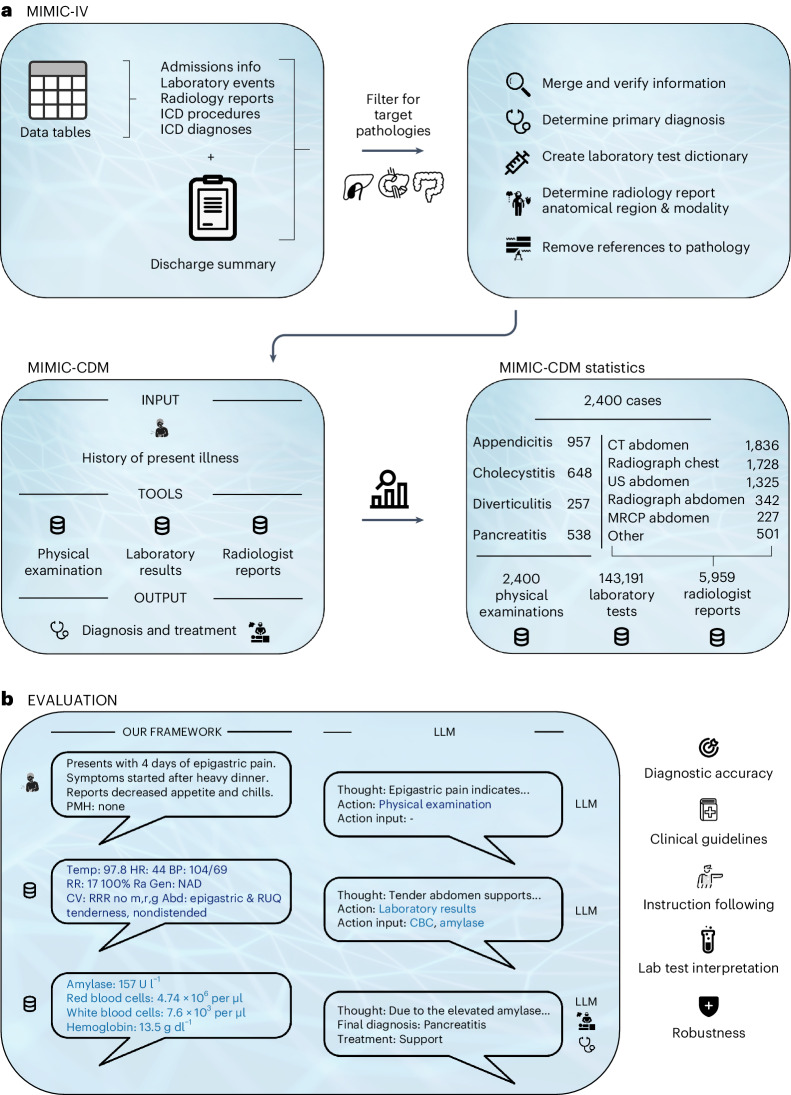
Overview of dataset creation and evaluation framework. a, To properly evaluate LLMs for clinical decision-making in realistic conditions, we created a curated dataset from real-world cases derived from the MIMIC-IV database, which contains comprehensive electronic health record data recorded during hospital admissions. b, Our evaluation framework reflects a realistic clinical setting and thoroughly evaluates LLMs across multiple criteria, including diagnostic accuracy, adherence to diagnostic and treatment guidelines, consistency in following instructions, ability to interpret laboratory results, and robustness to changes in instruction, information quantity and information order. ICD, International Classification of Diseases; CT, computed tomography; US, ultrasound; MRCP, magnetic resonance cholangiopancreatography.
